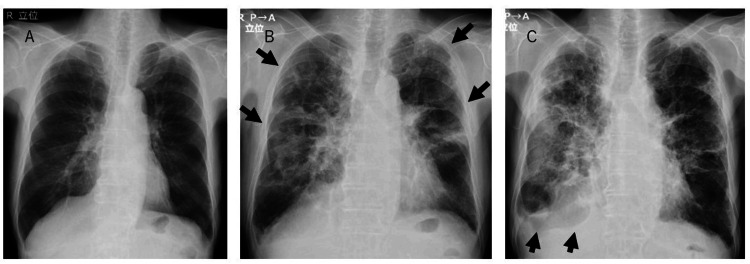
Serial changes on chest radiography. (A) Initial chest X-ray findings in a patient with COVID-19. (B) Five months after COVID-19 infection, bilateral infiltrates developed (arrows). (C) Eight months later, the infiltrates had progressed to involve the entire right lung field with associated pleural effusion (arrows). COVID-19, coronavirus disease 2019
